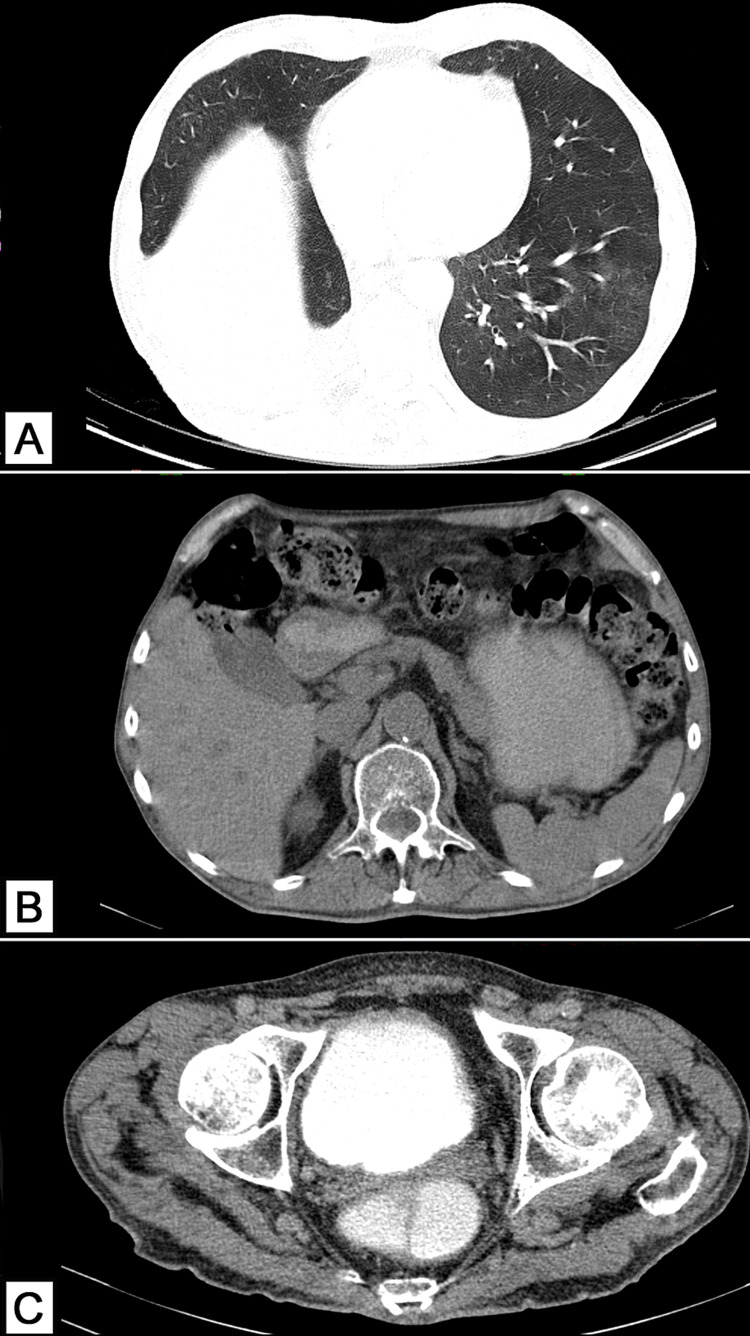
CT scan for recurrence and metastases detection (A) Axial CT scan (pulmonary window) is compatible with the patient’s history of right inferior pulmonary lobectomy; additionally, no masses or nodules are detected on either lung. (B) Normal abdominal CT scan with no signs of metastasis. (C) Normal pelvic CT scan with no signs of metastasis.
